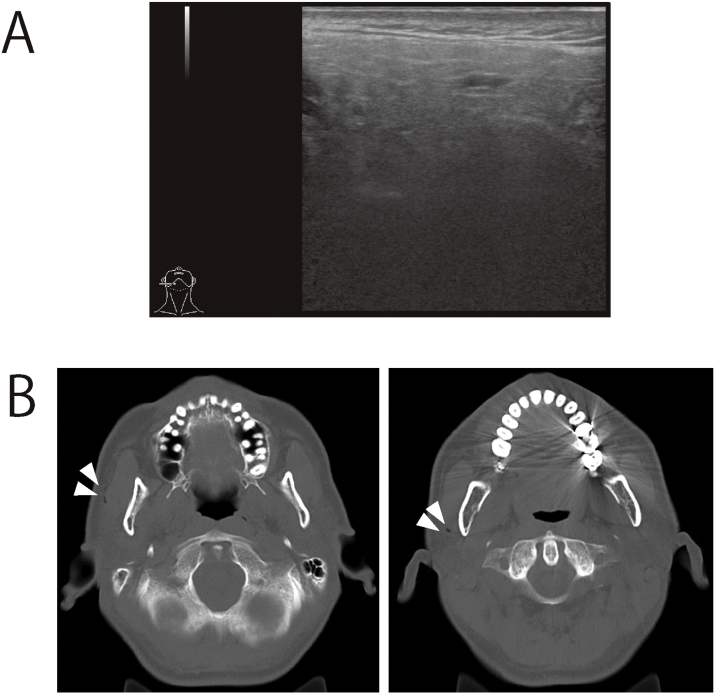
Imaging examination of a patient with pneumoparotid. (A) Ultrasound images. There were no obvious abnormal findings. (B) Gas infiltration was detected in the right parotid gland (white arrows).
